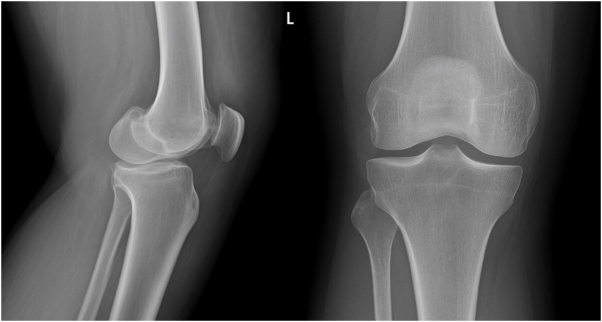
Legs’ front view and side view radiography immediately after trauma.
